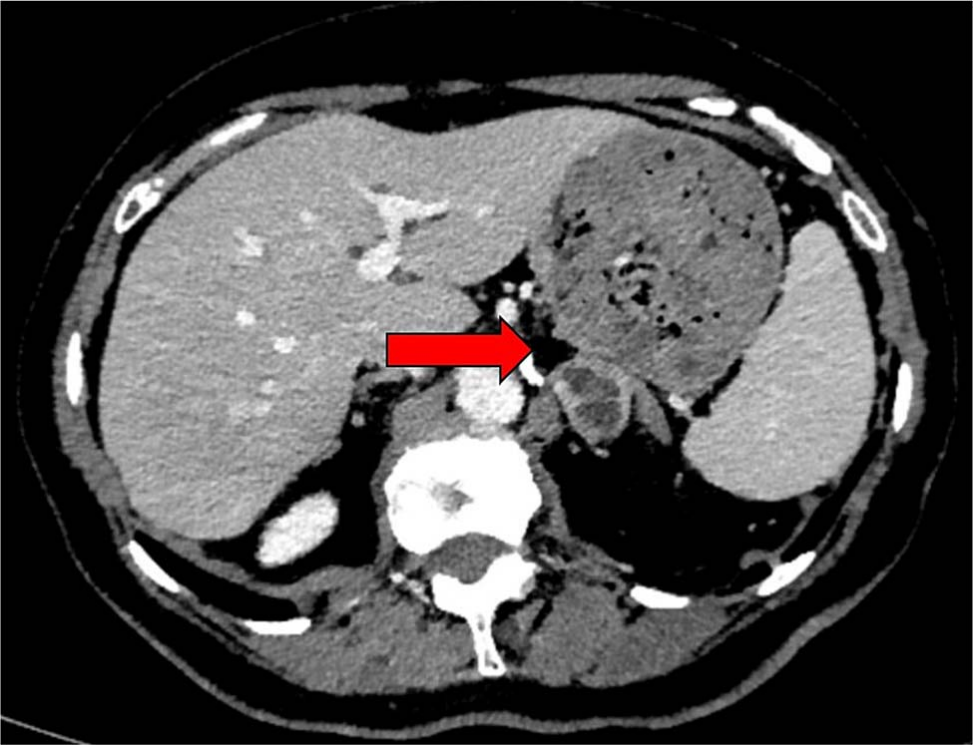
Preoperative axial CT image in 2024 showing an indeterminate left adrenal lesion measuring 28 × 26 × 13 mm, with heterogenous postcontrast enhancement and internal cystic change (indicated by arrow). Absolute and relative washout were calculated at 48.3% and 23.3%, respectively.
